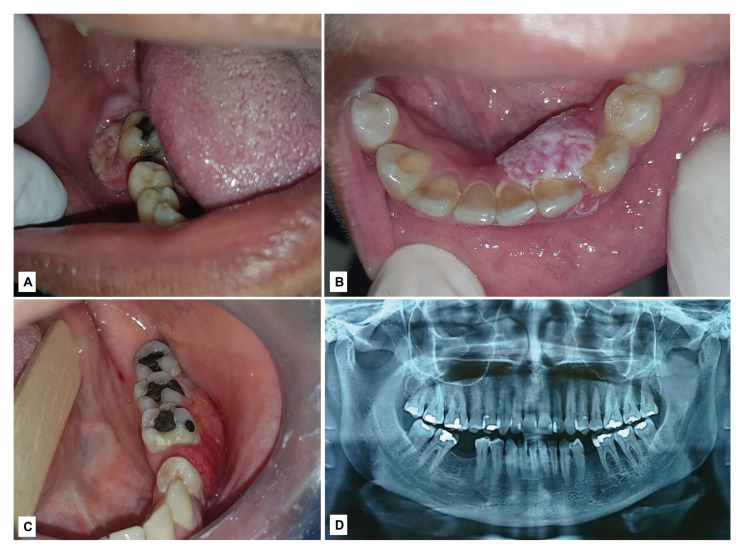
Clinical presentations of gingival squamous cell carcinoma. A - 53-year-old male patient, with an ulcerated lesion with indurated and raised margins in the gingiva of right mandibular second molar. B- 28-year-old female patient with a reddish-white nodule, with papillary surface, involving the buccal and lingual mandibular anterior gingiva at right. C- 42-year-old female patient with a reddish swelling in the left posterior mandibular gingiva, appearing with a granular surface and focal areas of telangiectasia. The first molar presented mobility and the patient was under treatment for periodontitis. D - The same patient of patient seen in C. Panoramic radiograph revealed a mandibular diffuse radiolucency in the region of the left first molar, which caused alveolar bone loss.
